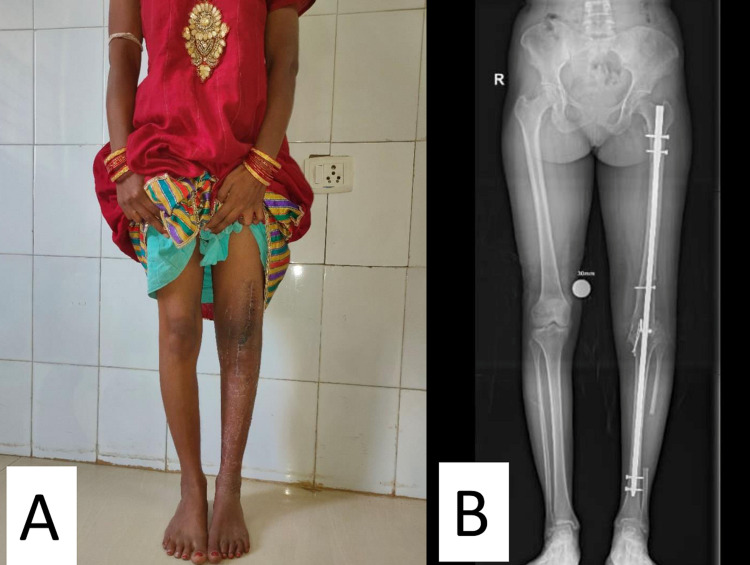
Clinical image and full length lower limb standing radiograph at one-year follow-up (A) Clinical image showing full weight-bearing. (B) Full-length lower limb radiograph showing long intramedullary locking nail in situ in the left lower limb with well callus formation at the arthrodesis site.
